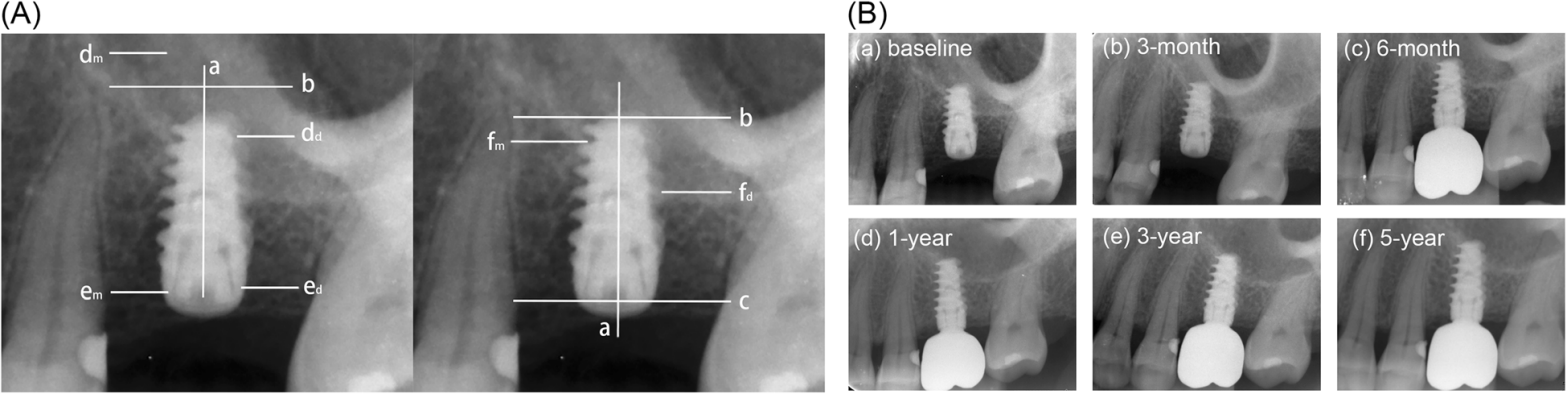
a Radiographic assessment. Reference lines were drawn as follows: (a) Implant longitudinal axis; (b) implant apex line: the most apical level of the implant, vertical to (a); (c) implant coronal line: the level of implant-to-abutment contact, vertical to (a); (d) Apical bone line: the most apical level of the new bone in the sinus, vertical to (a), at the mesial site (d_m_) and at the distal site (d_d_); (e) crest bone line: the most coronal level of bone-to-implant contact, vertical to (a), at the mesial site (e_m_) and at the distal site (e_d_); (f) Sinus floor line: the most coronal level of sinus floor cortical bone, vertical to (a) , at the mesial site (f_m_) and at the distal site (f_d_). b Endo-sinus bone remodeling after non-grafting OSFE
